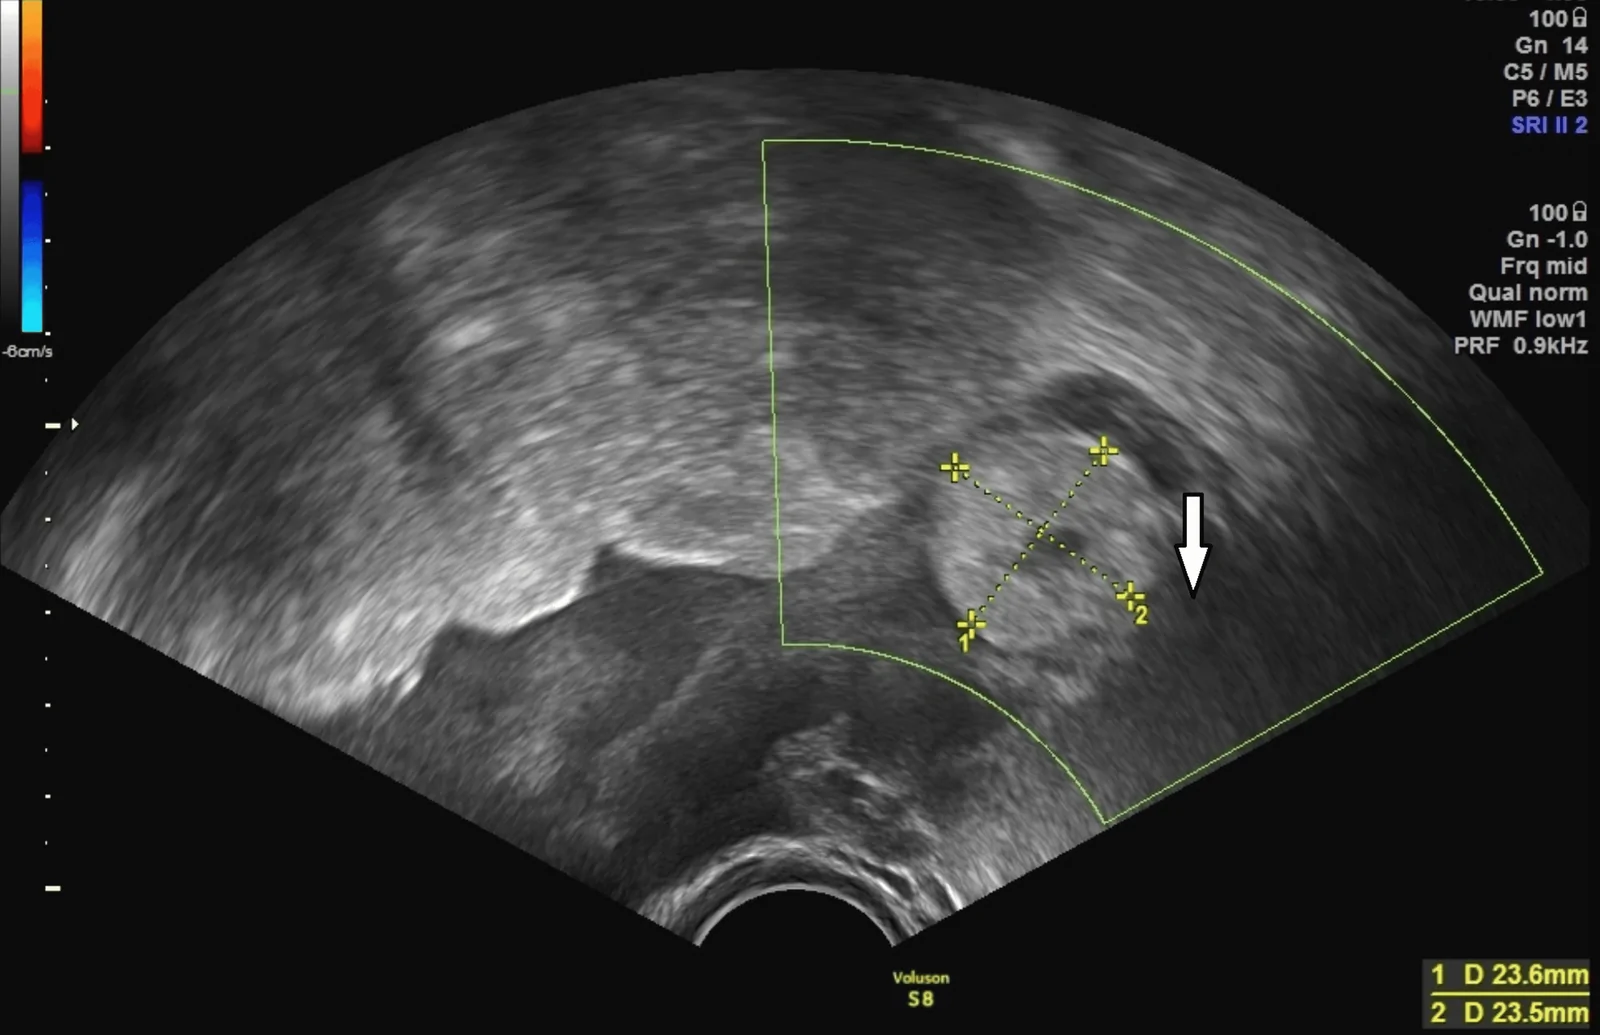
Transvaginal ultrasonography demonstrating a 23.6 × 23.5 mm left interstitial gestational mass adjacent to the uterine cavity. The white arrow indicates the left uterine horn.
